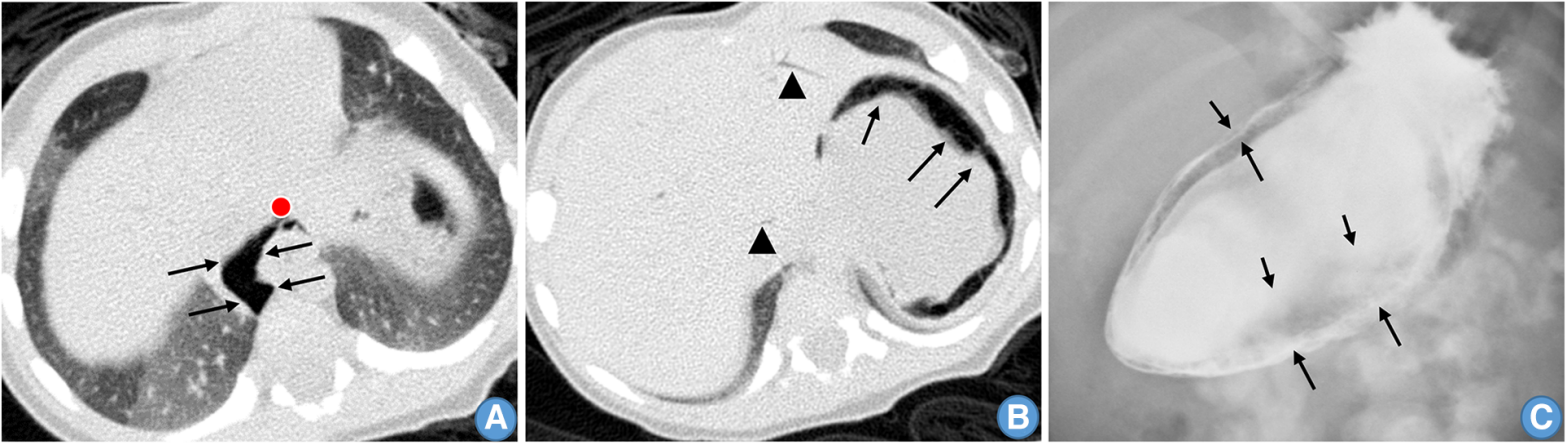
(A) Gas around the esophagus. (B) Gas is present in the stomach wall (arrows) and portal vein (triangles). (C) Imaging (x-ray) shows a large amount of gas in the stomach wall (arrows).
